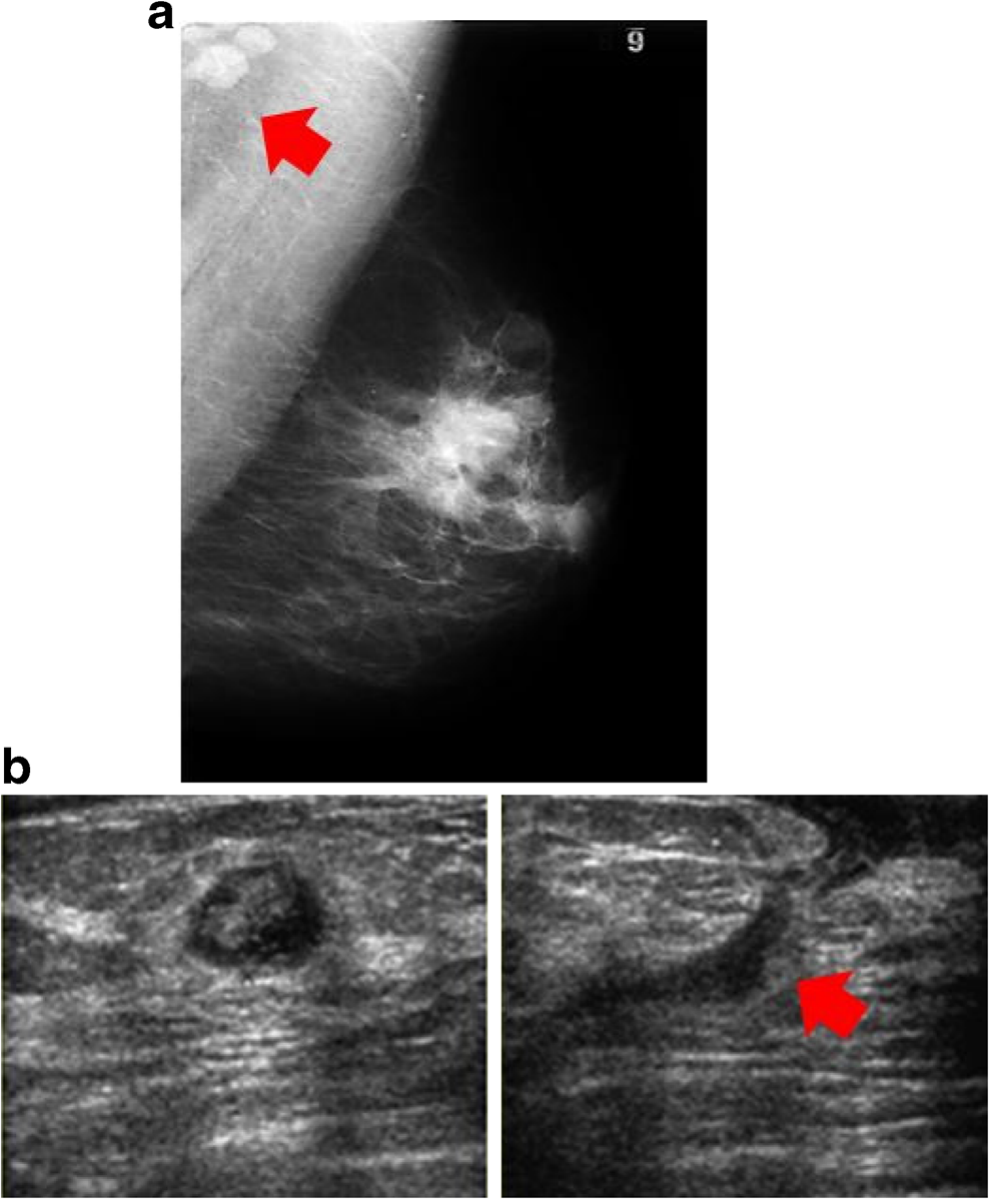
Infectious cause: *Mycobacterium fortuitum*. A 32-year-old woman had a new tender, palpable mass in the left breast. Biopsy showed granulomas, and purulent drainage from the needle tract was positive for *M. fortuitum*. Treatment with antibiotics, based on the sensitivity of the organism, led to resolution of the infection. a Left mediolateral-oblique view showed multiple masses with prominent left axillary lymph nodes (*red arrow*) and periareolar skin thickening. b Ultrasound showed a complex, hypoechoic fluid collection (*left*) with sinus tract extending to skin (*red arrow*) (*right*)
